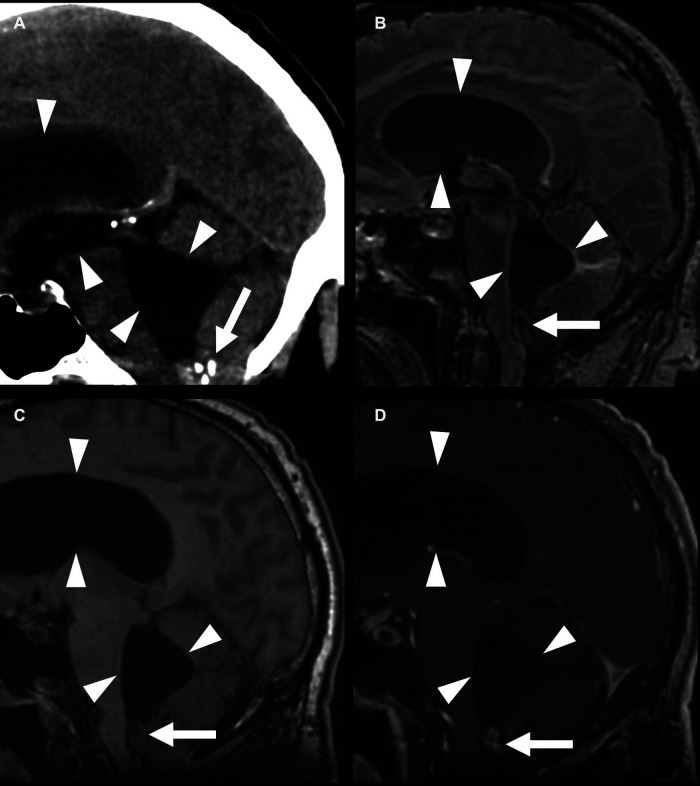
Fourth-ventricle subependymoma in a 54-year-old man. CT shows a partially calcified mass at the inferior aspect of the fourth ventricle (A) (arrows), with an associated supratentorial and infratentorial hydrocephalus (arrowheads). This mass is FLAIR (B) and T1 (C) isointense, with post-contrast enhancement (D). The central T1 and FLAIR hypointensity is due to susceptibility due to calcifications.
